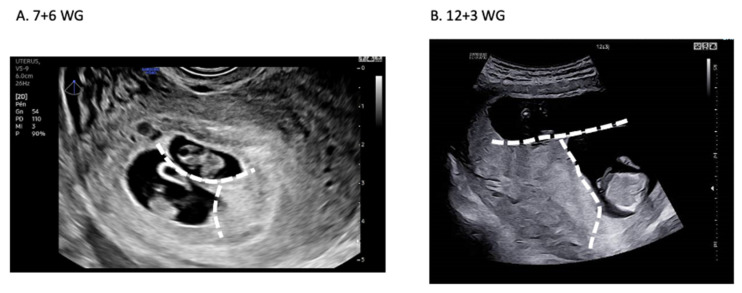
Ultrasound assessment of dichorionic diamniotic twin pregnancy. (A) First ultrasound scan at 7+6 WG showing two amniotic gestational sacs with a “lambda” sign referring to the triangular appearance of the chorion (dotted white line). (B) Confirmation of the dichorionic diamniotic twin pregnancy at 12+3 WG.
